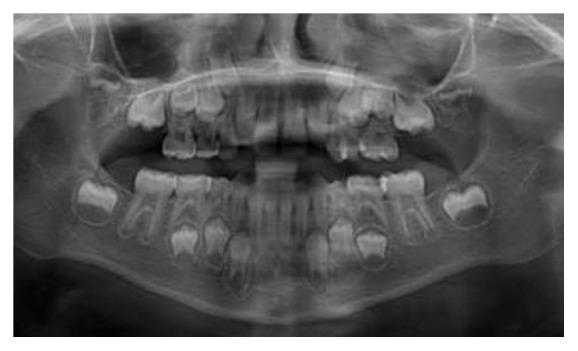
Panoramic X-ray photograph at the age of eight years showing agenesis of 16 and 26.
